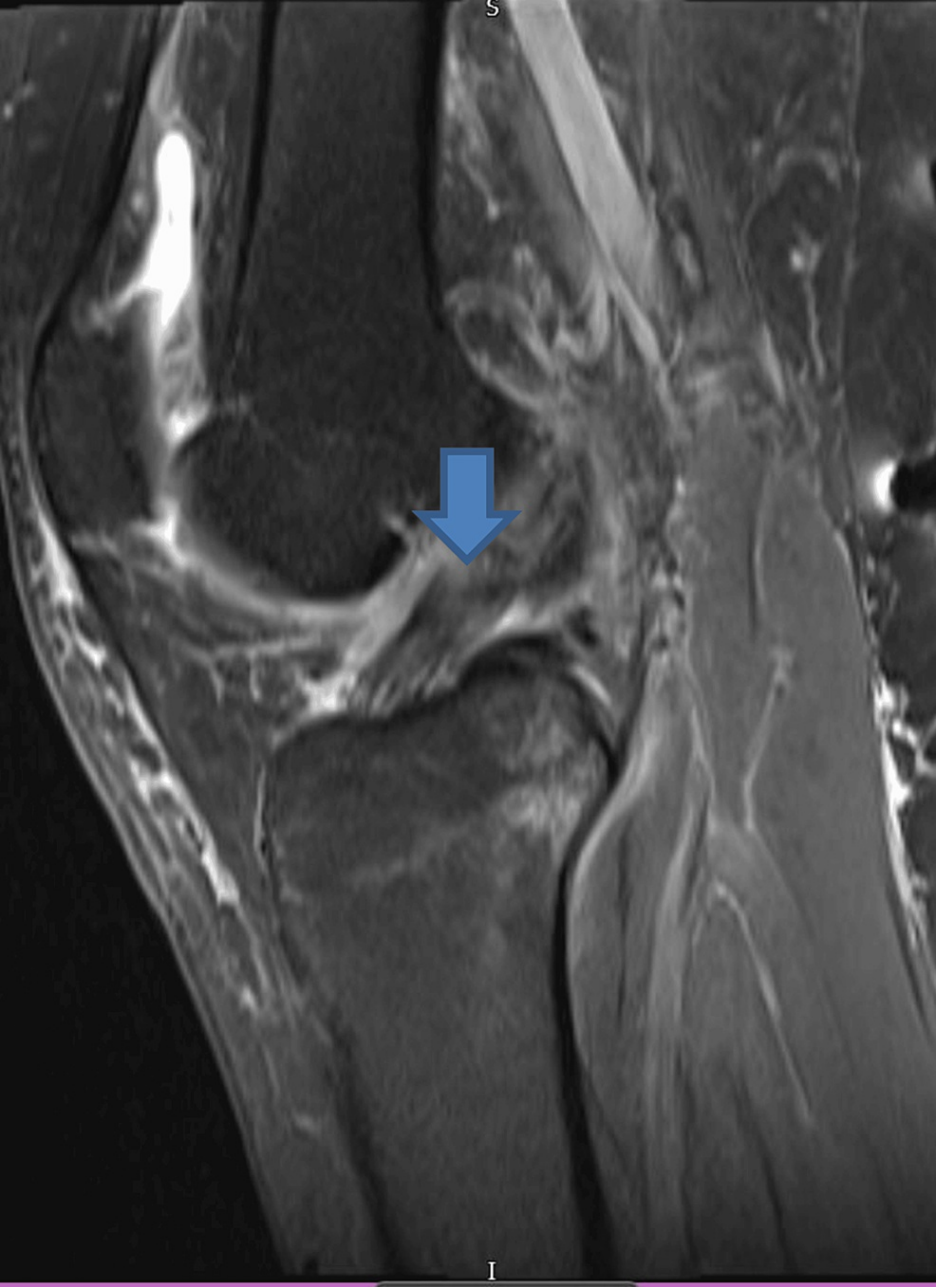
Magnetic resonance imaging of the left knee shows anterior cruciate ligament injury (blue arrow)
